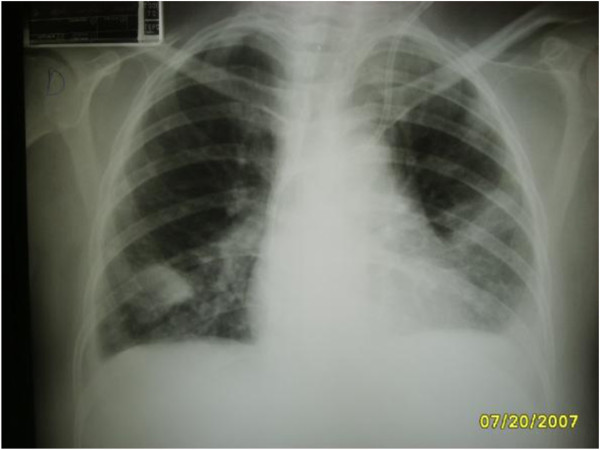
Image of chest radiography with areas of opacity and an image of double-lumen CVC for HD implanted in the left jugular vein.
